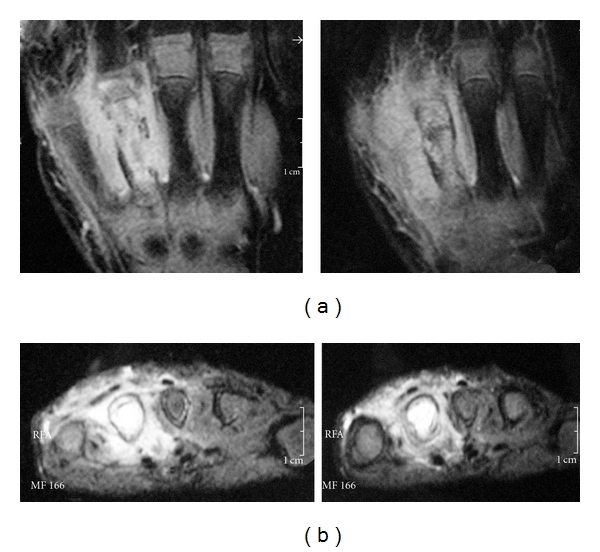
MRI findings, diaphyseal and physeal destruction, and bone oedema and soft tissue oedema.
